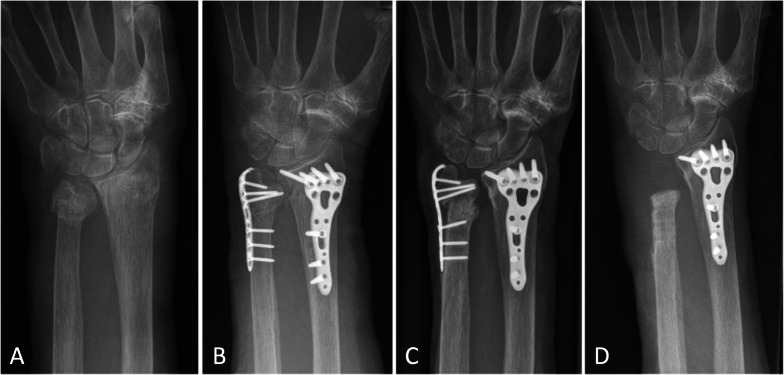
Nonunion after ulna plate osteosynthesis in an old patient. A Preoperative radiograph of a 93-year-old female presenting a Biyani type IV left distal ulna fracture combined with a type C distal radius fracture. B Immediate postoperative radiograph showing left wrist osteosynthesis with ulna hook plate and radius volar plate. C One-year postoperative radiograph showing ulna and radius fracture nonunion. D Radiograph after ulna head resection (Darrach) due to ulna nonunion
